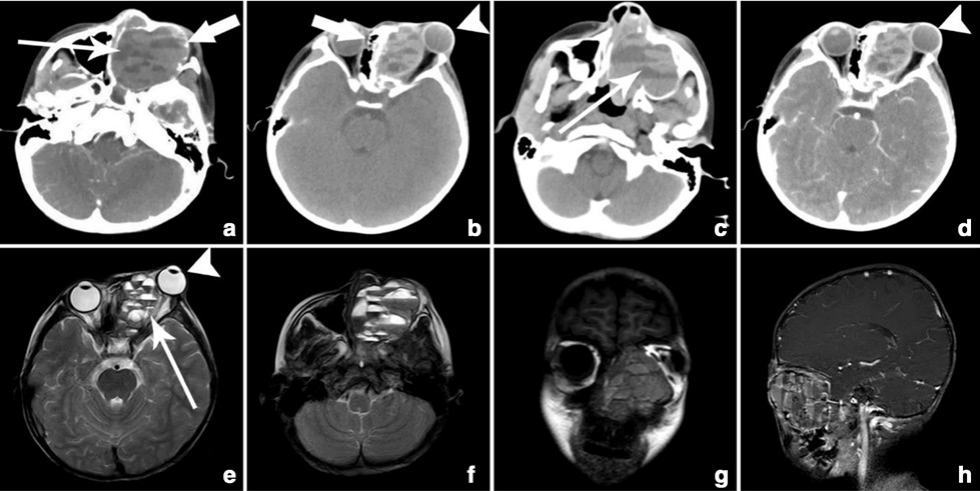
(a–h) Case 3: axial non-enhanced CT images (a, b) and contrast enhanced CT images (c, d), axial MR *T*_2_WI (e, f) and coronal MR *T*_1_WI (g) and contrast enhanced sagittal MR *T*_1_WI (h) demonstrating uneven density soft tissue mass arising from maxillary bone and expanded into the maxillary sinus, left orbital cavity, paranasal sinuses and within nasal cavity resulting in protrusion of left eyeball (arrow head), destruction of adjacent bony structures (thick arrow), multiple fluid levels (thin arrow) and deviated nasal septum towards the right side. *T*_1_WI, *T*_1_ weighted image; *T*_2_WI, *T*_2_weighted image
